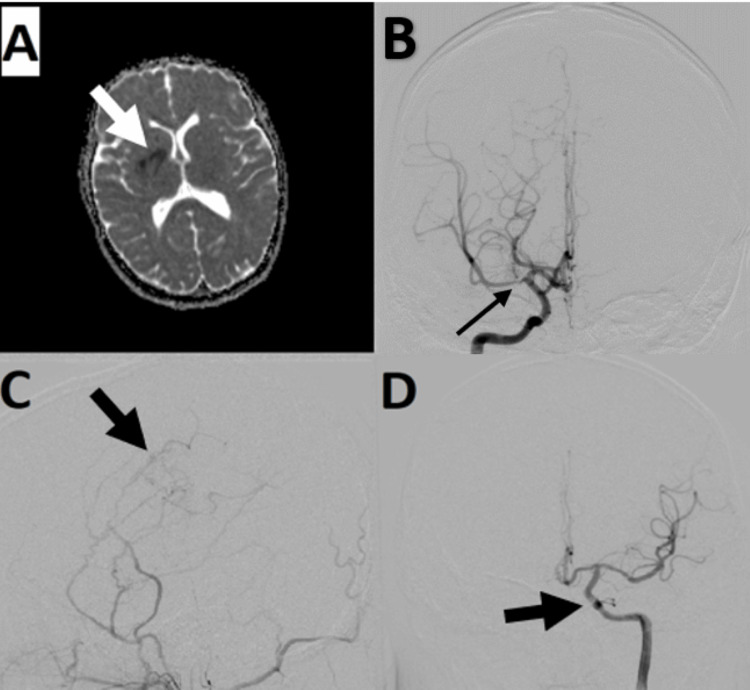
Cranial imaging before the onset of left-sided symptoms 1a: Axial apparent diffusion coefficient (ADC) MRI from 2013 showing hypointensity (arrow) in the right basal ganglia, consistent with an infarct. 1b: AP right ICA angiogram showing narrowing of the right MCA (arrow) seven months after the initial stroke. 1c: Lateral right external carotid artery angiogram showing revascularization (arrow) after EDAS in 2019. 1d: AP left ICA angiogram showing normal caliber of the left ICA in 2013.
